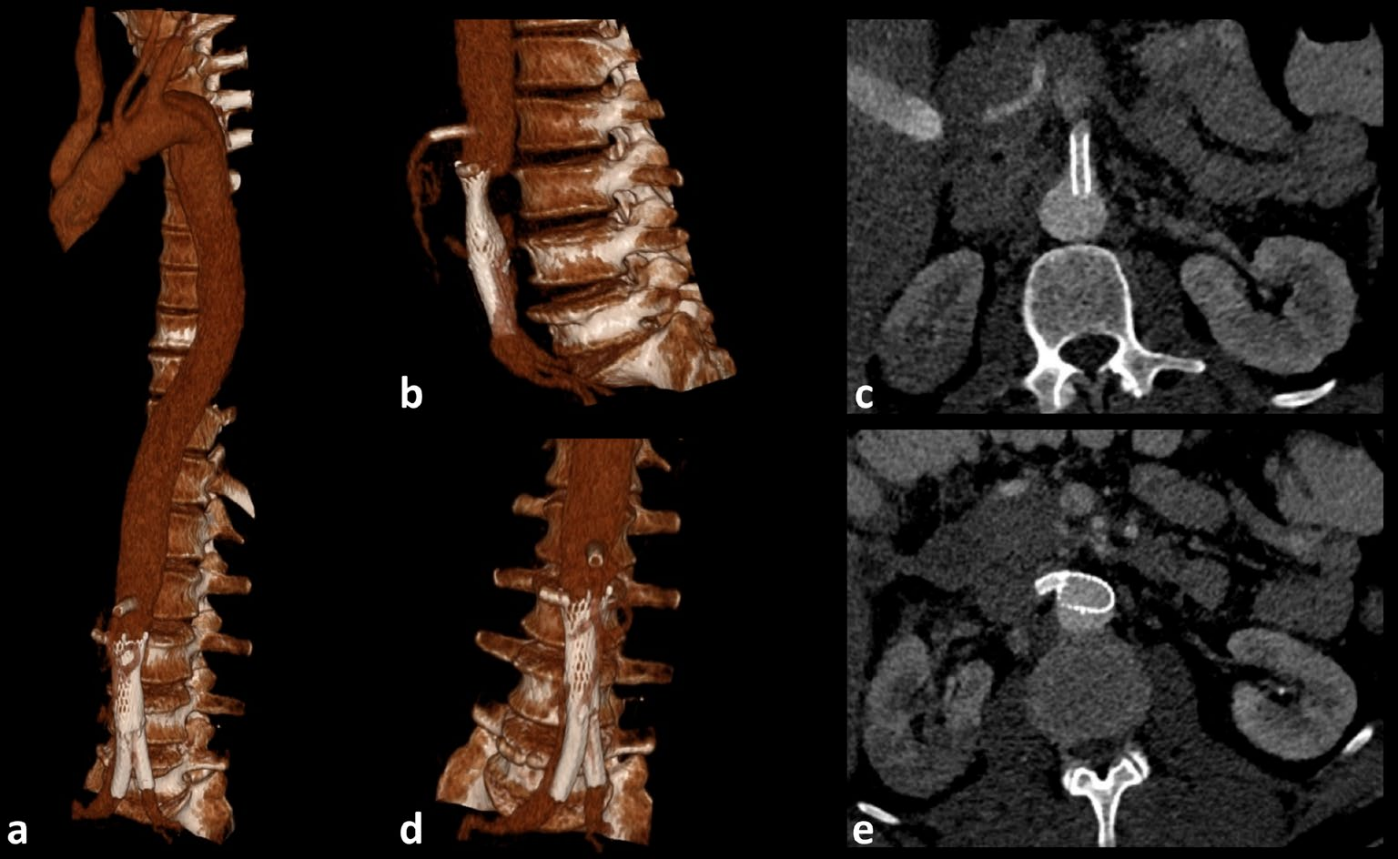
Final outcome of type‐A aortic dissection repair (a, b, d) 3D reconstructions showing the final results of surgical and interventional treatment for the aortic type‐A dissection. The procedures included a David procedure, partial aortic arch replacement, stenting of the superior mesenteric artery (SMA), stenting of the right renal artery, and implantation of two iliac stent‐grafts with remaining stenosis of the infrarenal aorta. (c, e) Axial abdominal computed tomography angiography demonstrating good opacification of the stents in the SMA (c) and the right renal artery (e). Additionally, partial infarction of the right kidney due to the initial dissection is visible.
